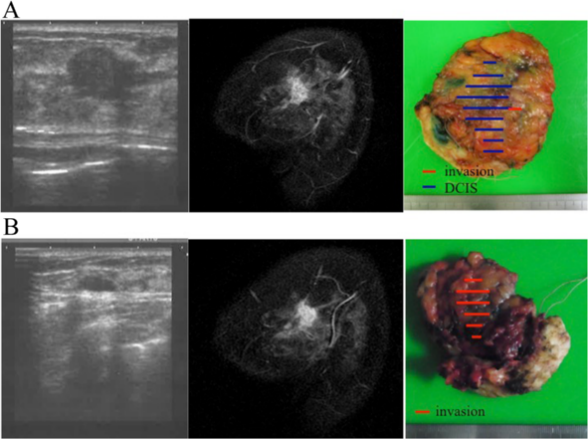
Ultrasound (US: *left panel*), magnetic resonance imaging (MRI: *center panel*), and pathological findings (*right panel*) of case 1 (a, *upper line*) and case 2 (b, *lower line*). US and MRI show a localized mass in each case. Partial mastectomy was performed prior to immediate reconstruction using a free dermal fat graft, which resulted in surgical margins that were negative for cancer in both cases (a, b, *right panel*)
